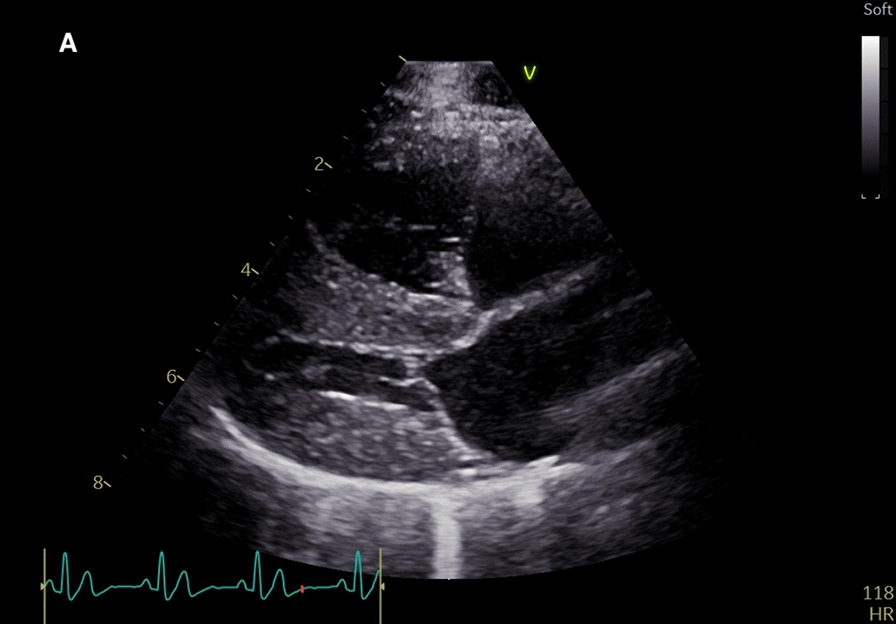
Echocardiography performed pre-treatment (A) from the right parasternal long axis four chamber view showing mild right chamber dilation, and from the short axis view showing mild interventricular septal flattening
